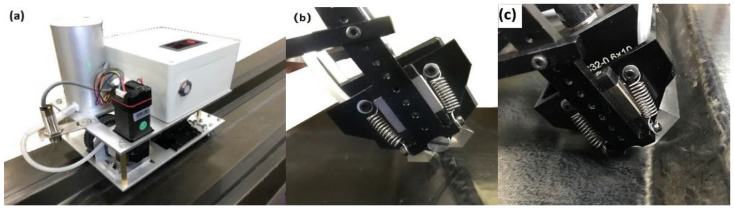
Non-destructive testing and sound field verification device on stringer through phased array ultrasonic technique: (a) Overall view; (b) Upper R-corner detector; (c) Lower R-corner detector.
